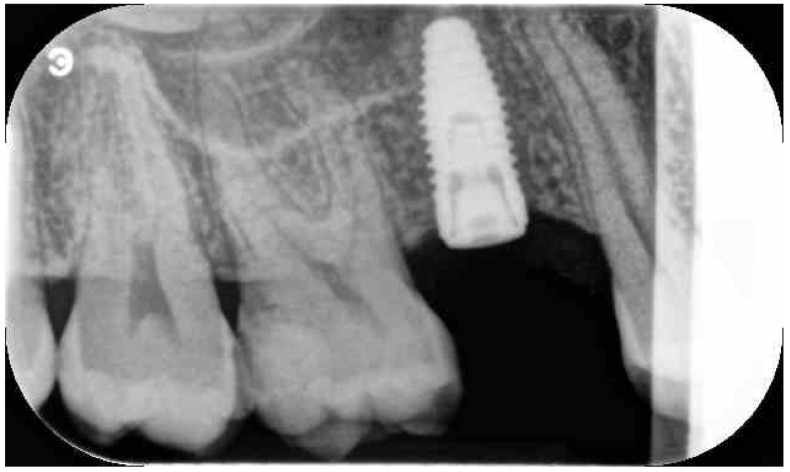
Periapical X-ray immediate after implant installation.
